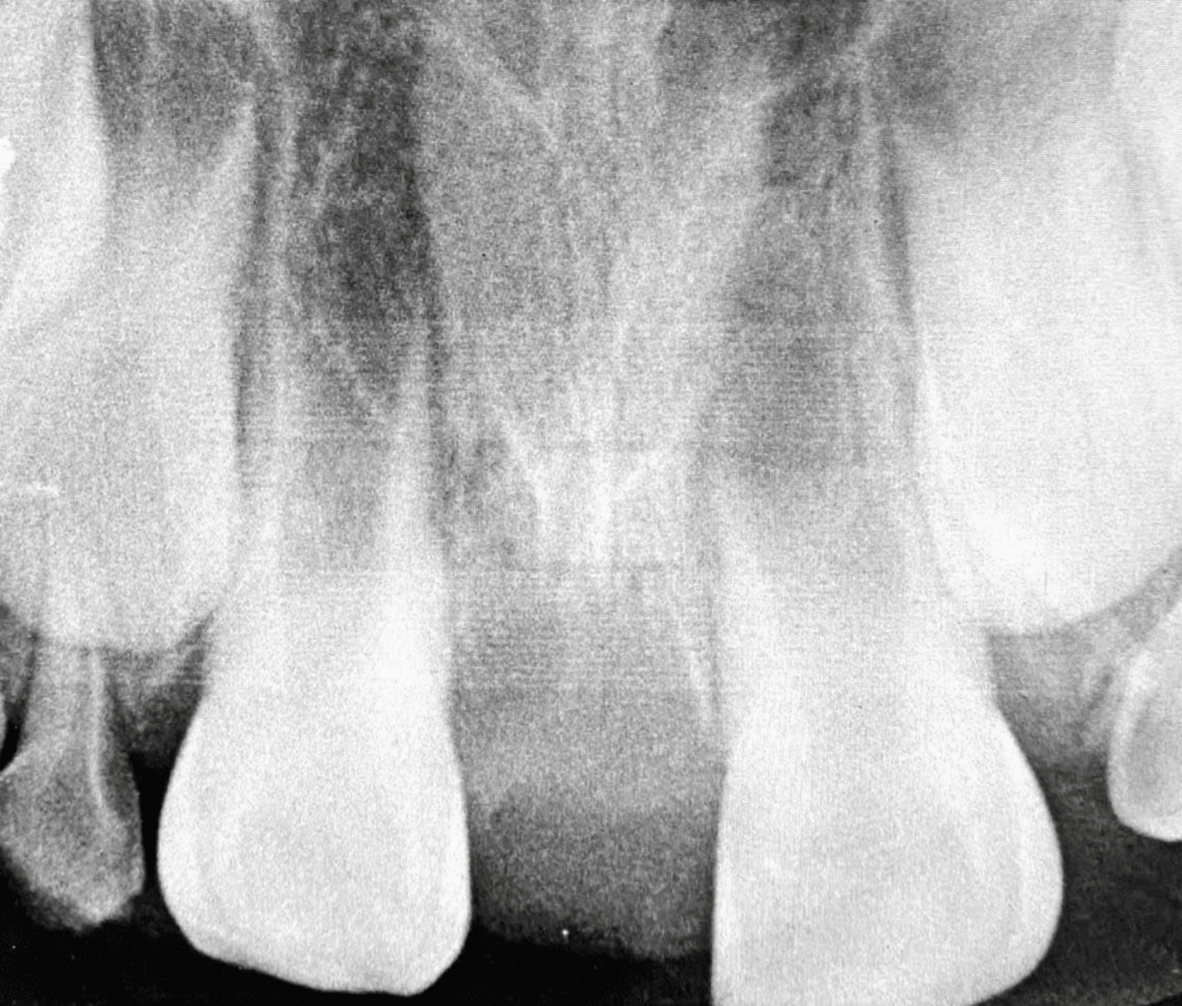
Periapical radiograph taken two weeks after surgery
